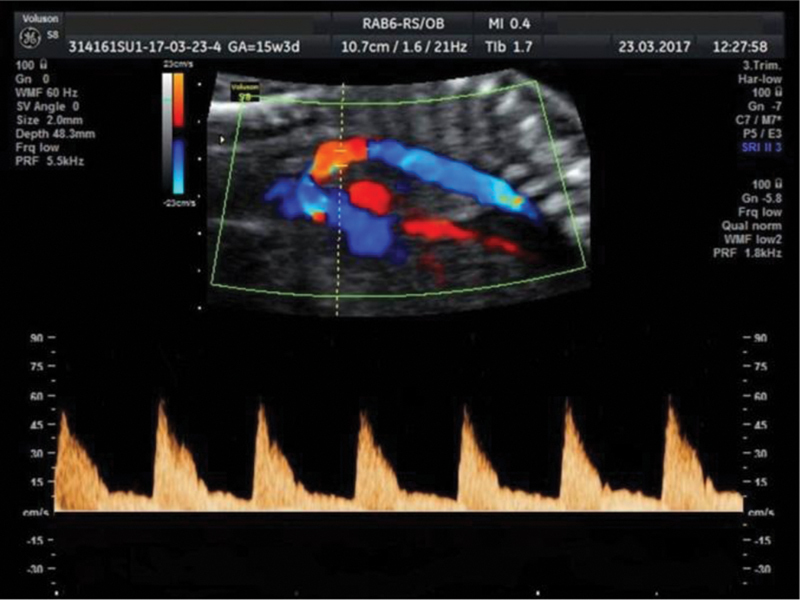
Aortic isthmus Doppler of a 15-week fetus showing a smooth and uniform deceleration without incisura.
